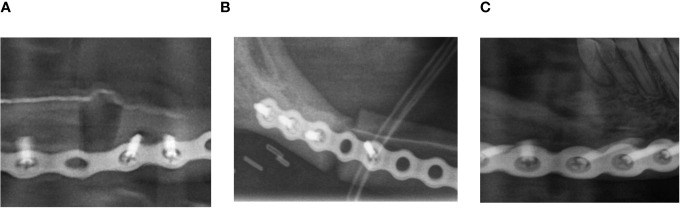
Example of evaluation of the initial contact point. (A) Example of no initial contact (radiographic gap >2 mm). (B) Example of moderate initial contact (radiographic gap ≤2 mm). (C) Example of good initial contact (no radiographic gap).
